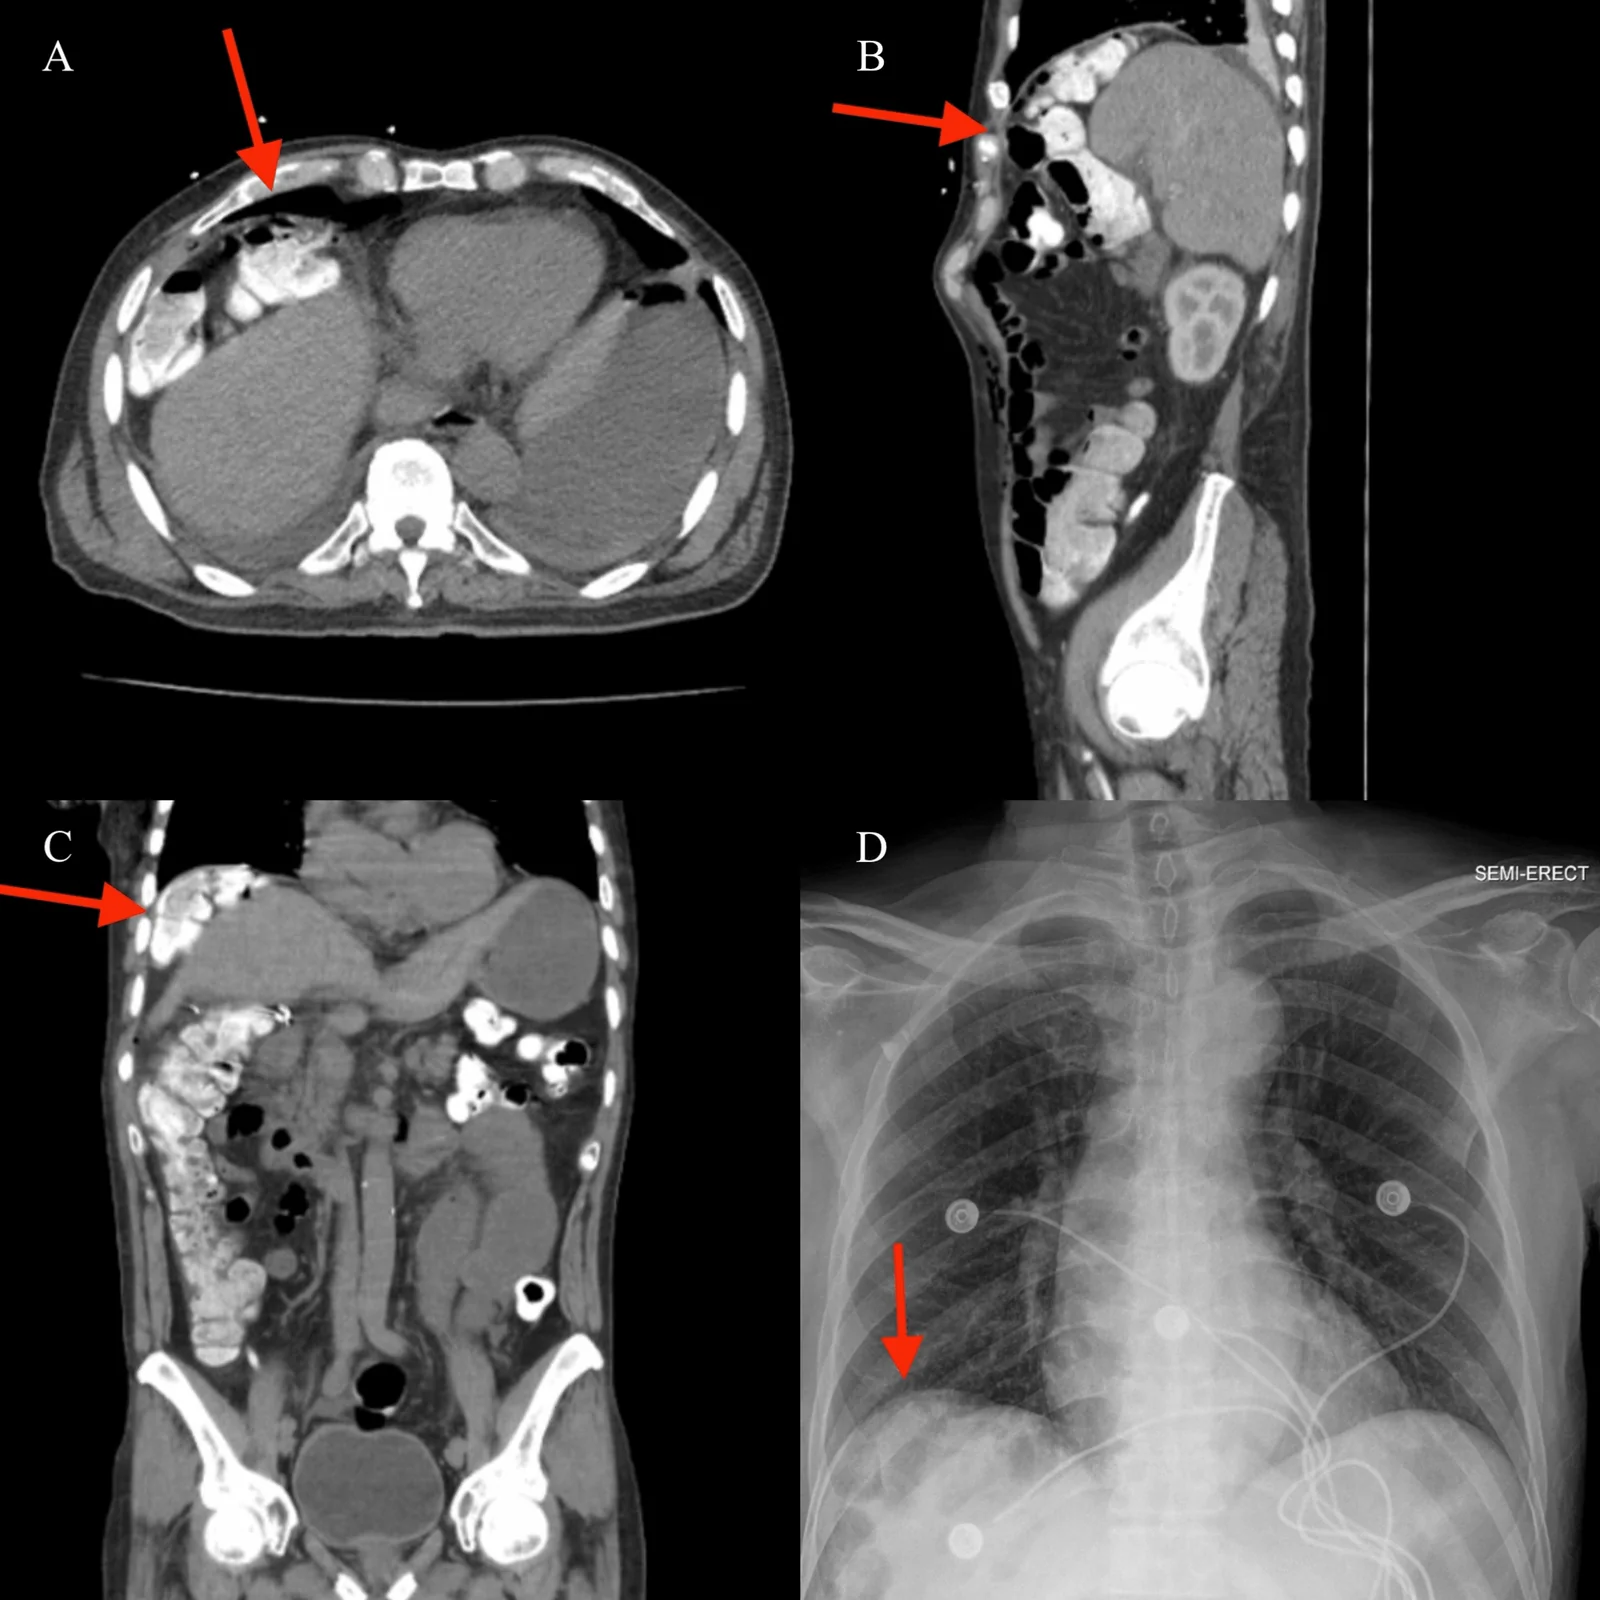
Imaging demonstrating the Chilaiditi sign. (A–C) Axial, sagittal, and coronal computed tomography images showing colonic interposition between the liver and right hemidiaphragm (red arrows). (D) Chest radiograph demonstrating subdiaphragmatic gas (red arrow) consistent with the Chilaiditi sign.
